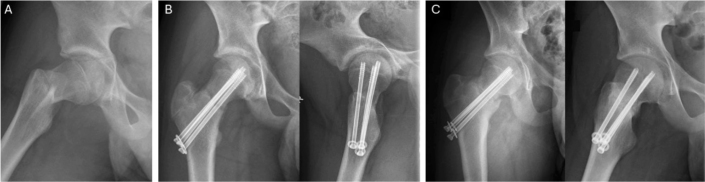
(A) Primary radiograph showing a dislocated Delbet II fracture, (B) fracture alignment following operation, and (C) non-union 7 months post-injury
